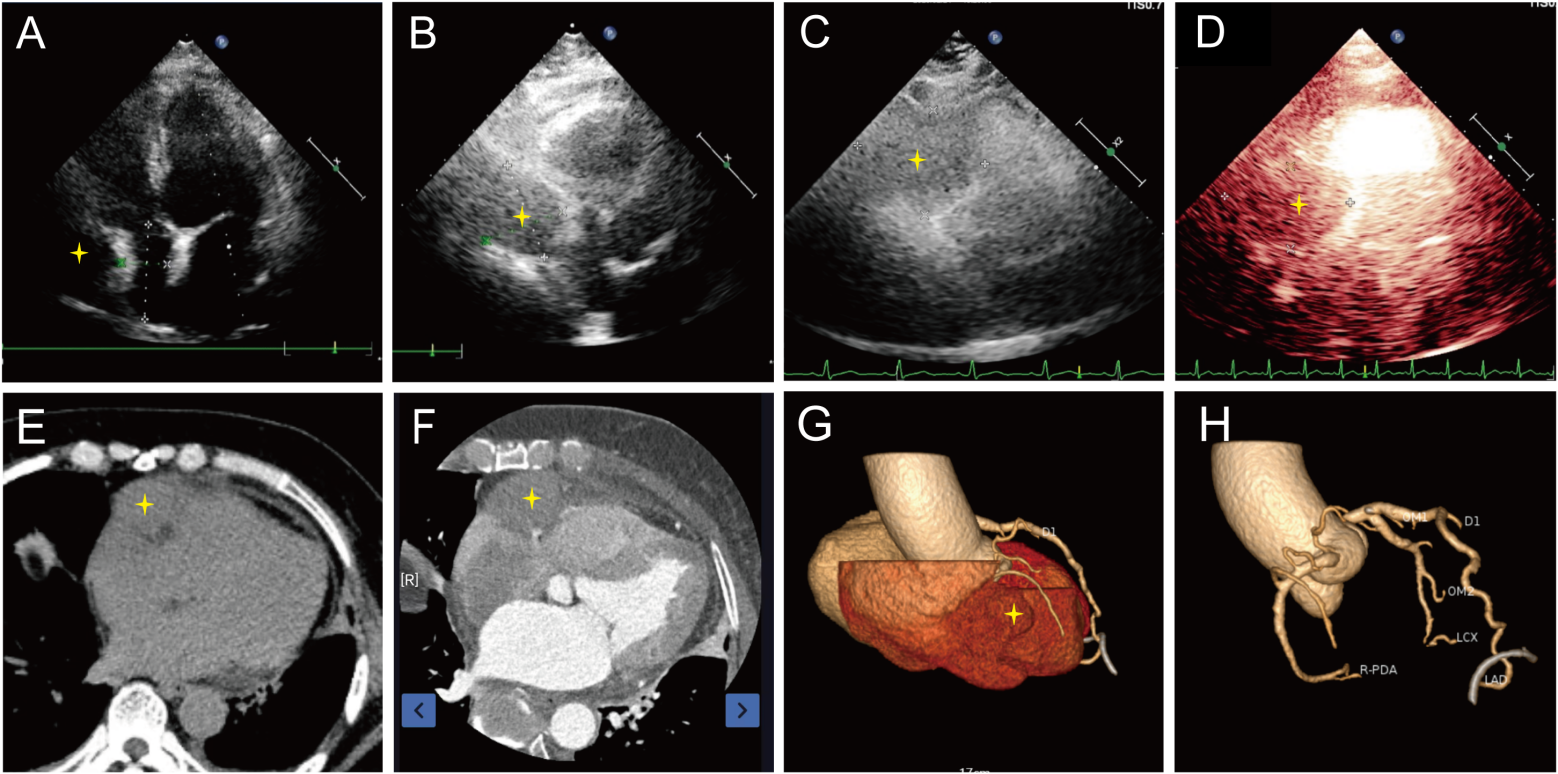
Preoperative imaging. Transthoracic echocardiography revealed a hypoechoic mass in the right atrioventricular groove, with regular contours and ill-defined borders, causing significant compression and deformation of the right atrium (A, B). Myocardial contrast echocardiography showed low perfusion within the mass (C, D). Contrast-enhanced computed tomography characterized the mass in the right atrioventricular groove (E) and highlighted its proximity to and compression of the adjacent right coronary artery (F). Three-dimensional reconstruction of the heart (G) and coronary arteries (H). Star: Primary cardiac lymphoma.
